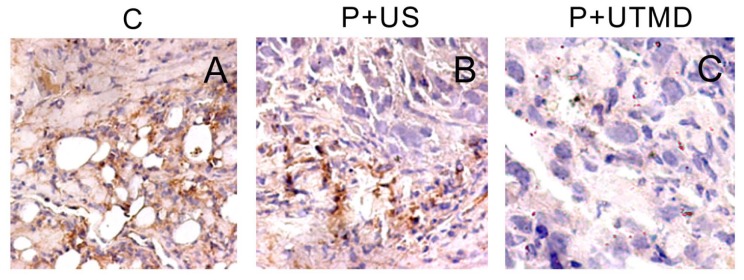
Comparison of CD34 expression in different groups (×400). C: control group; P + US: plasmid + ultrasound irradiation group; P + UTMD: plasmid + microbubble + ultrasound irradiation group.
